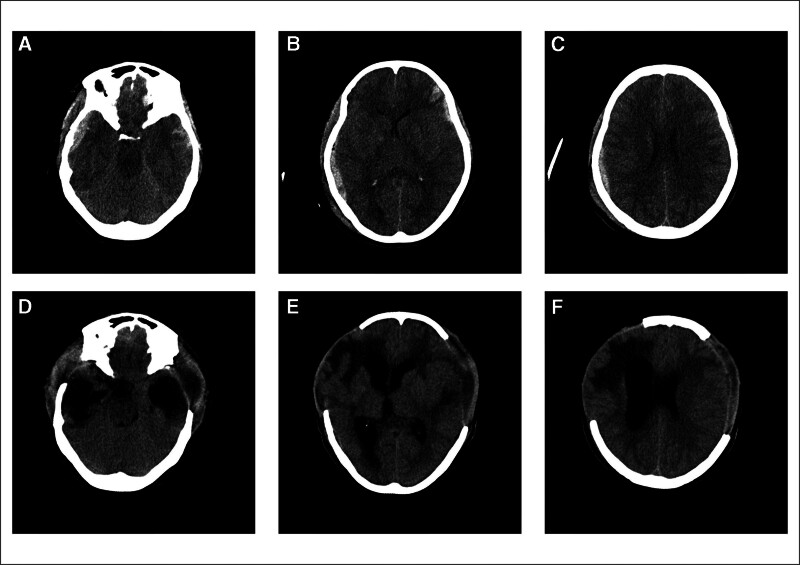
Case 1: A 41-year-old female with TBI in November 2020. Preoperative CT (A–C) showed the patient had bilateral temporal subdural hematoma with high intracranial pressure, circumferential cistern and lateral ventricle were compressed, the sulci disappeared. reexamination CT (D–F) of 10 days after surgery revealed multiple low-density lesions in the intracranial and enlarged ventricles. TBI = traumatic brain injury.
